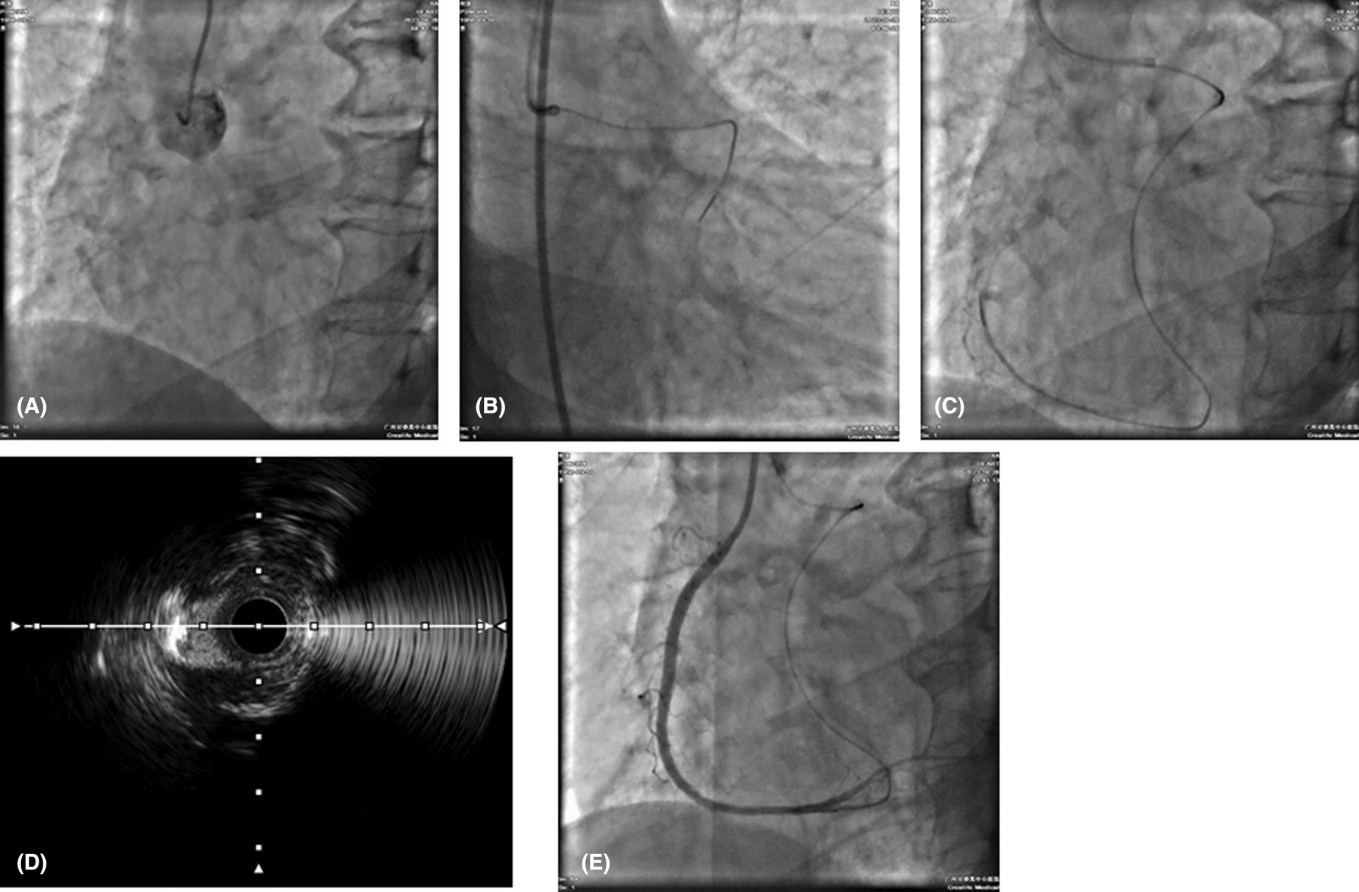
Multiple examination images. (A) Stumpless chronic total occlusion (CTO). (B) Retrograde approach is established via Grade 2 collateral connection. (C) SUOH03 guidewire advances through Grade 2 collateral connection to cross the occlusion at the right coronary artery (RCA) opening to reach the ascending aorta. (D) Intravascular ultrasound (IVUS) findings indicating CTO. (E) Dilation of the obstructed vessel.
